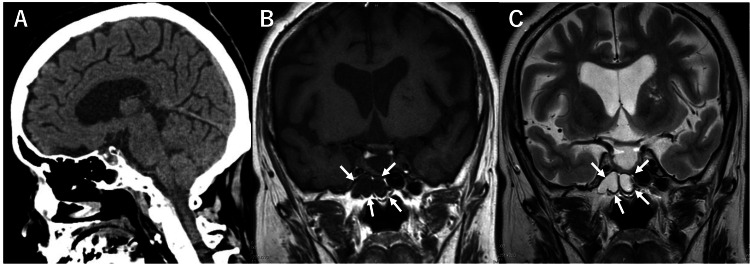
Preoperative CT and MRI findings (case 1) (A) Preoperative sagittal CT showed no obvious bone defects at the skull base. (B) The coronal T1-weighted MRI image showed a low signal within the sphenoid sinus. In contrast (C), the coronal T2-weighted MRI image showed a high signal, suggesting the presence of fluid accumulation within the sphenoid sinus (white arrow). CT, computed tomography; MRI, magnetic resonance imaging
